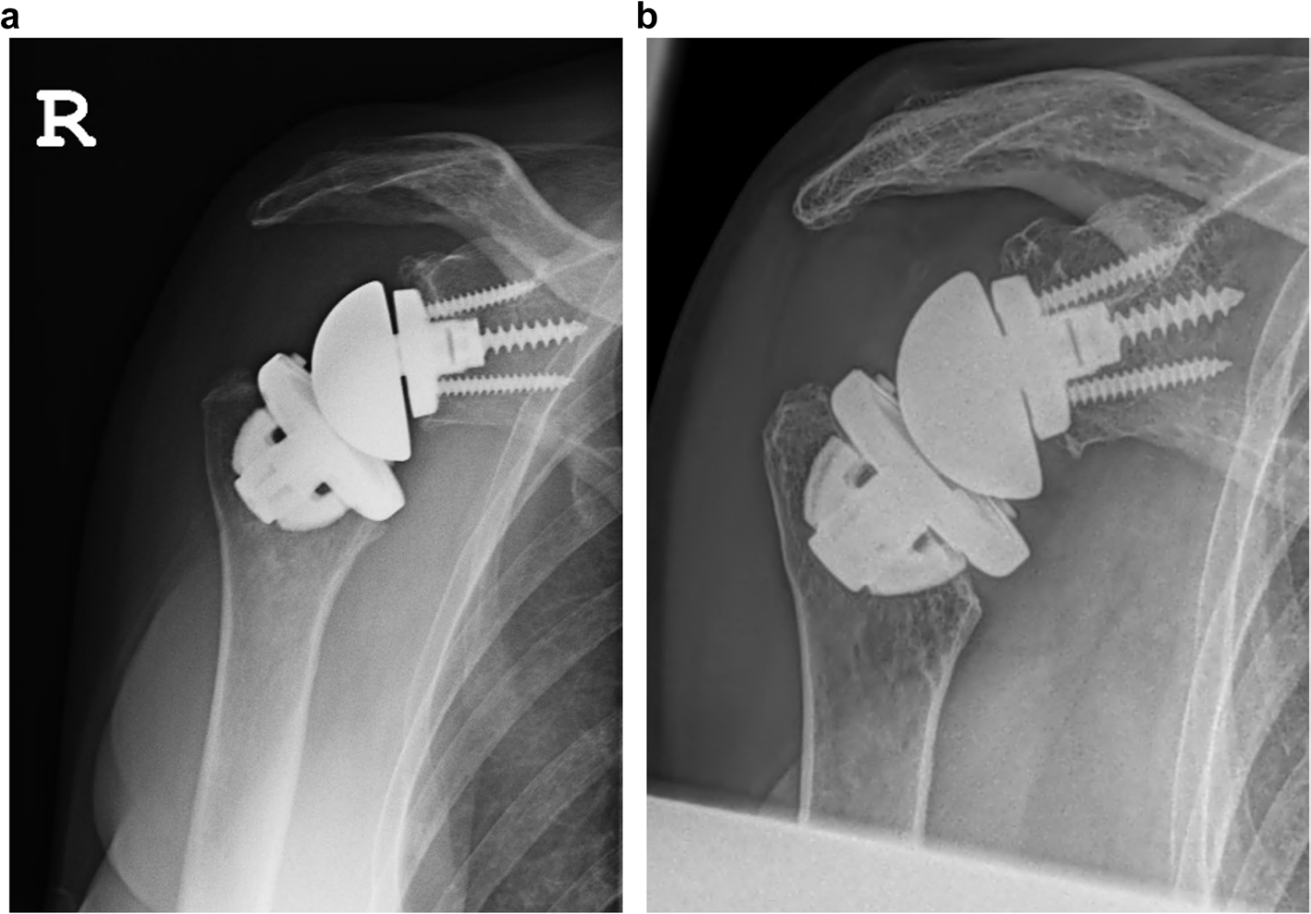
X-ray imaging postoperatively (a) and at the 128-month follow-up (b) showing secondary resorption in the calcar region
